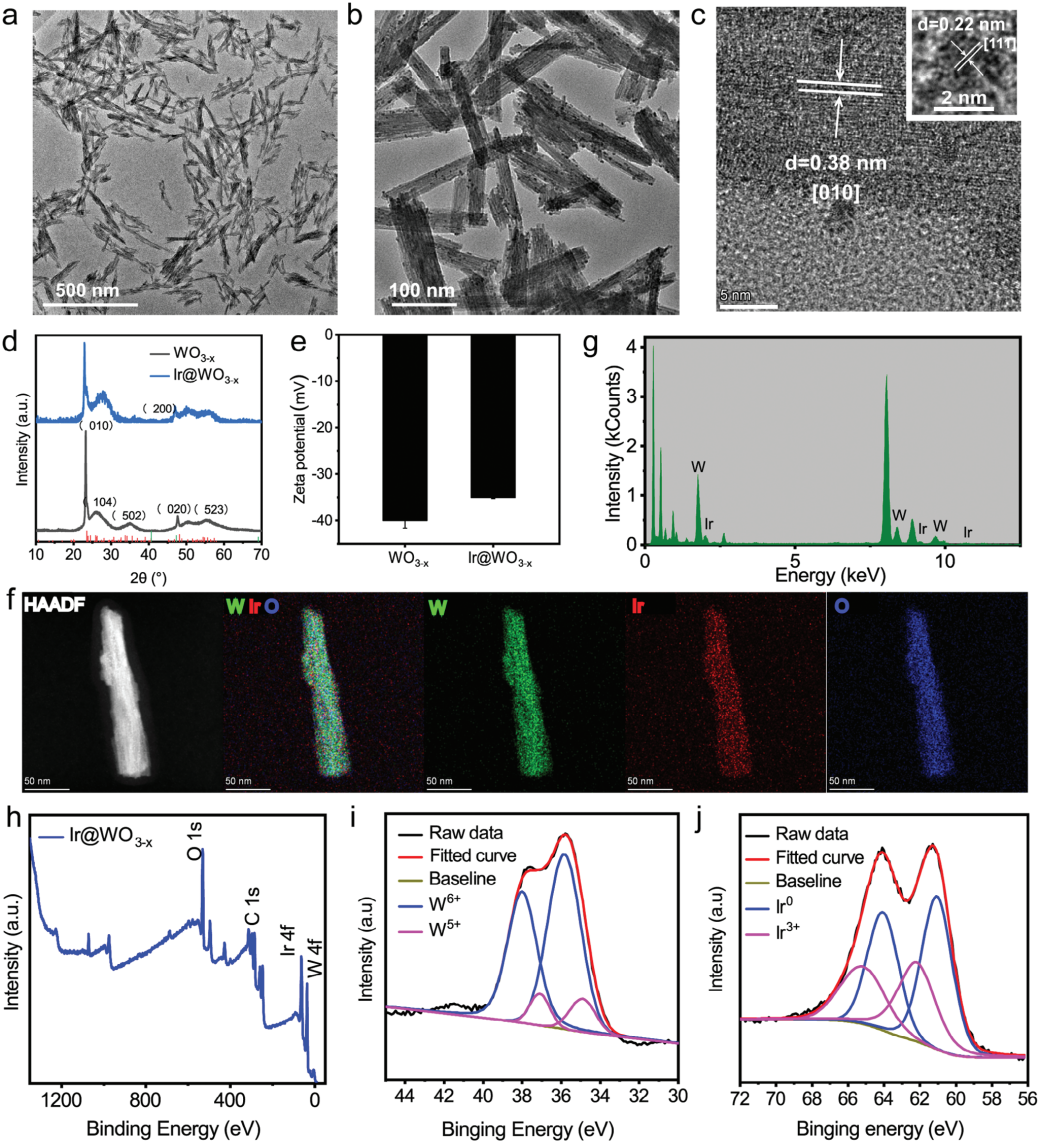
Nanoreactor characterization. a) TEM image of WO_3−x_ after ultrasonic fracture. b) TEM image of Ir@WO_3−x_. c) High‐resolution TEM image of Ir@WO_3−x_. d) XRD pattern of Ir@WO_3−x_. e) Zeta potential of Ir@WO_3−x_ (mean ± SD, *n =* 3). f) HAADF‐STEM image and element mapping of Ir@WO_3−x_. g) EDS spectra of Ir@WO_3−x_. h) XPS spectra of Ir@WO_3−x_ nanoreactors. XPS spectra of W 4f i) and Ir 4f j) core levels in Ir@WO_3−x_ nanoreactors.
